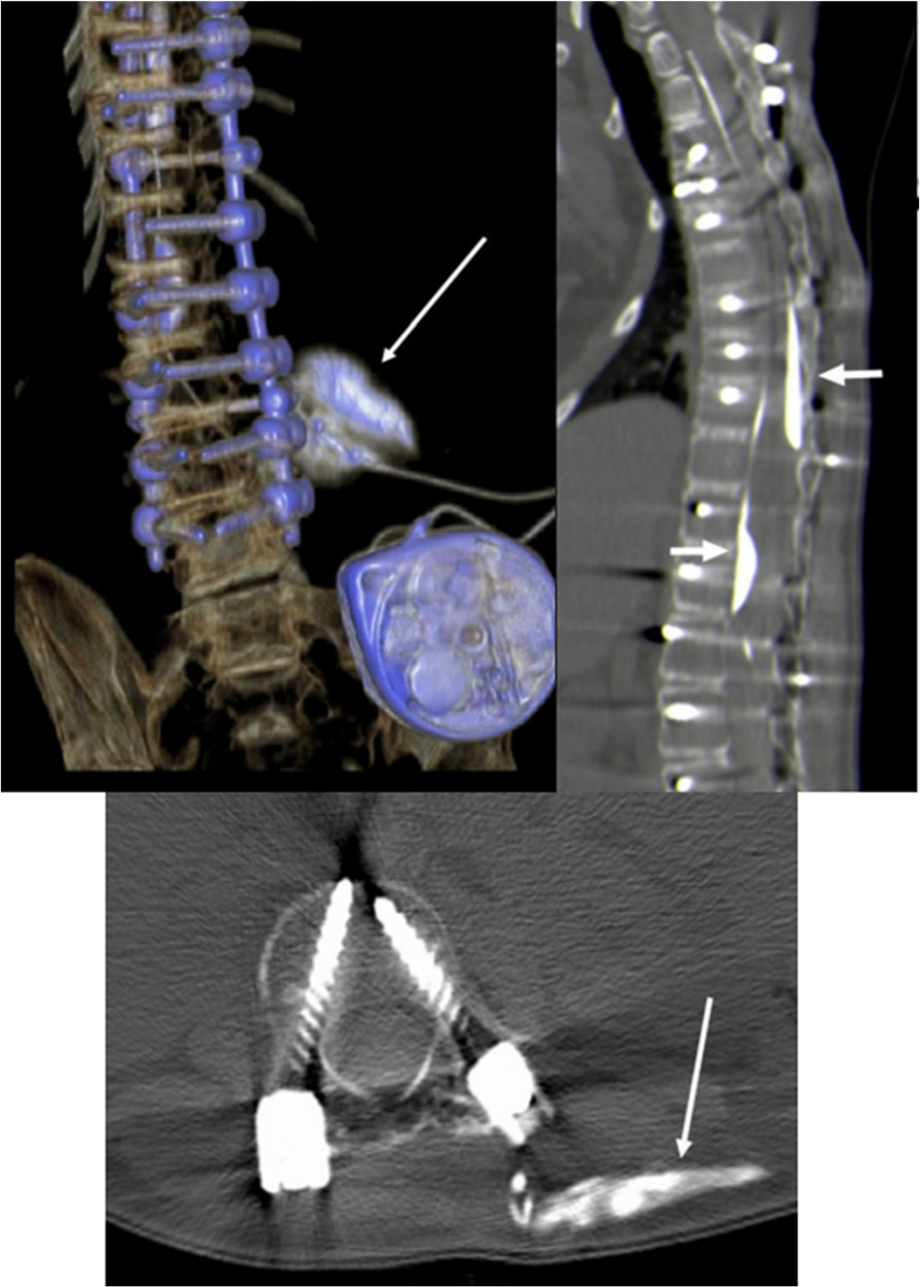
Case 3, CT shows catheter rupture: spiral CT of the spine after injection of the baclofen pump with 15 ml solutrast: evidence of a subcutaneous contrast medium extravasation as a sign of a tube rupture dorsal to L4 (long white arrow). In addition, most likely misalignment of the catheter tip with two subdural contrast agent deposits (short white arrows). Only minute amounts of contrast medium are displayed intrathecally
